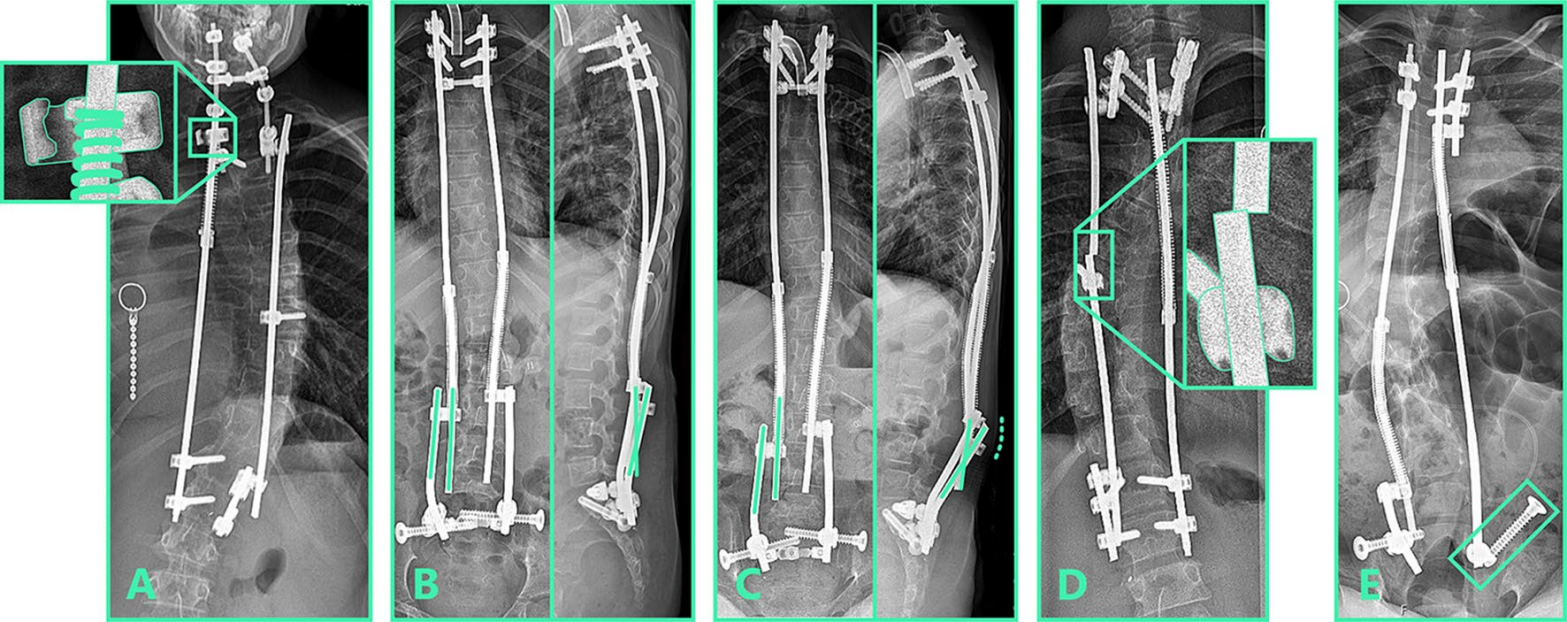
Spring distraction system complications. Examples of spring distraction system complications. a Fatigue failure of sliding side-to-side connector. b Post-operative radiograph showing the angle that the rods make in the coronal and sagittal plane. c After several years of follow-up, distraction caused kyphosis between the sliding and the static rod that resulted in prominence (dashed line). d Rod fracture near the apical screw. e Distal anchor failure. The iliosacral screw backed out of its original iliosacral trajectory
